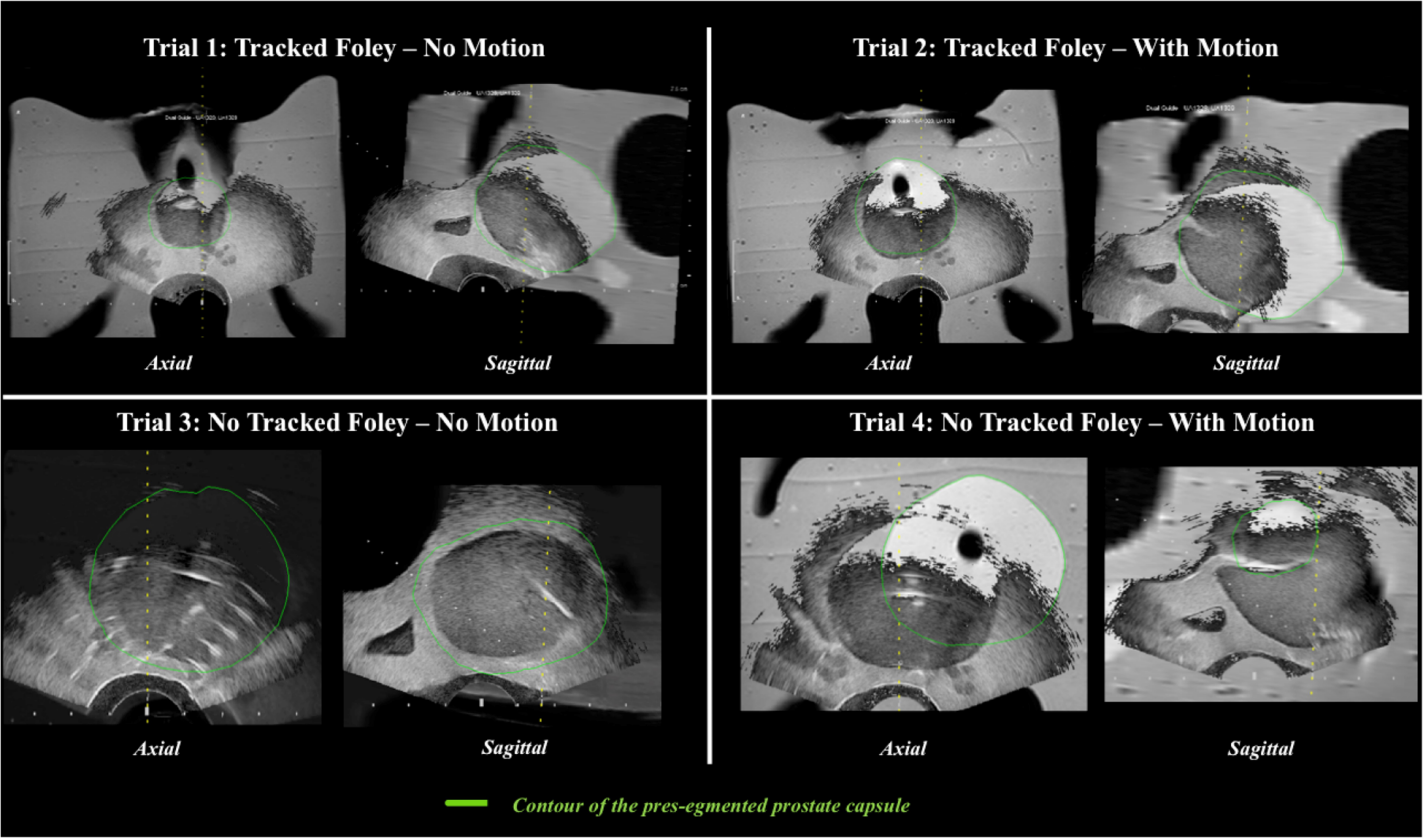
Intra-experimental screen captures from trials 1 to 4: magnetic resonance imaging (MRI)/ultrasound (US) fusion images, displayed with variable windowing blending MRI and US. Note trial 3 images showing the US predominantly on the left and more MRI on the right. The green contour is the pre-segmented prostate capsule from the pre-procedural MRI
